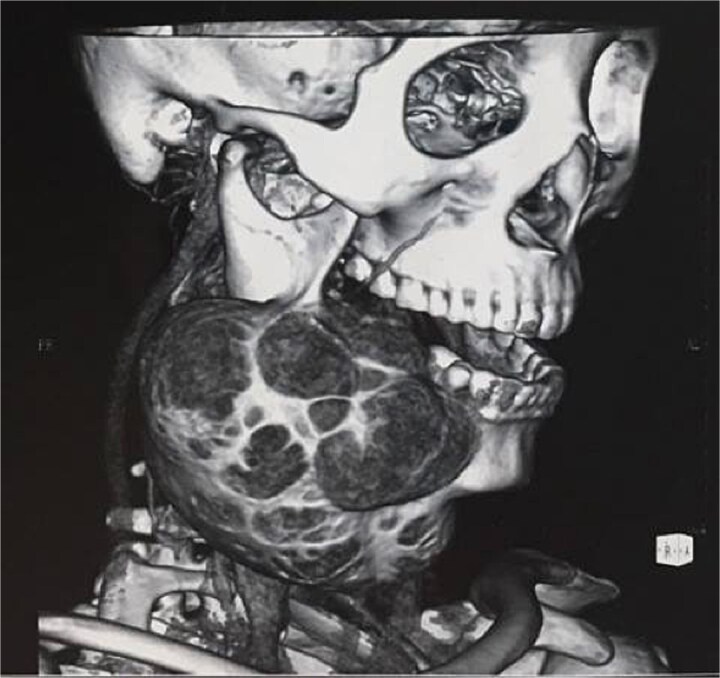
Preoperative CT. Three-dimensional reconstruction demonstrating extent of mandibular involvement.
